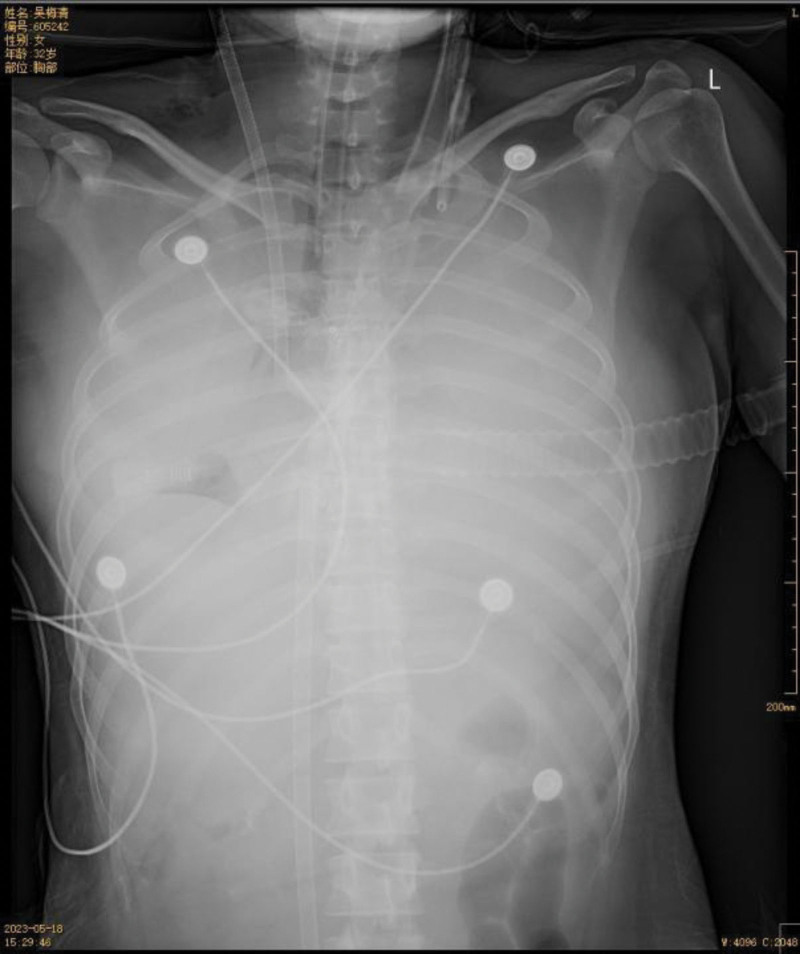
On the afternoon of May 18th, chest X-ray showed decreased volume and increased density in the right lung, while the left lung exhibited a large patchy high-density shadow resembling “white lung” appearance.
